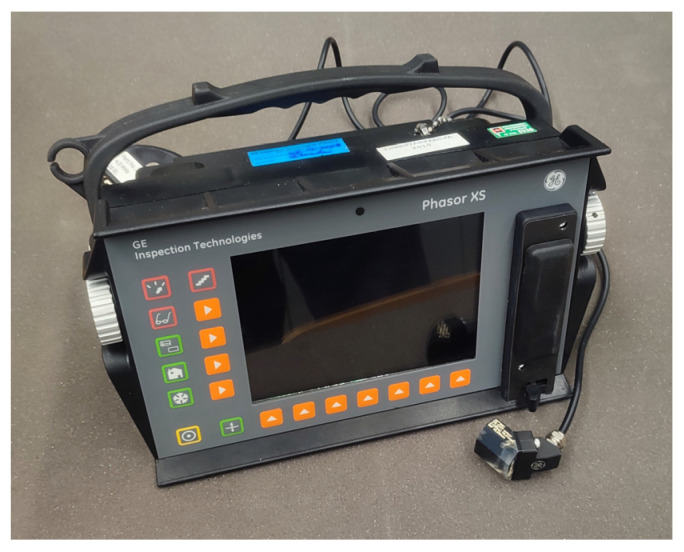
Ultrasonic flaw detector.
